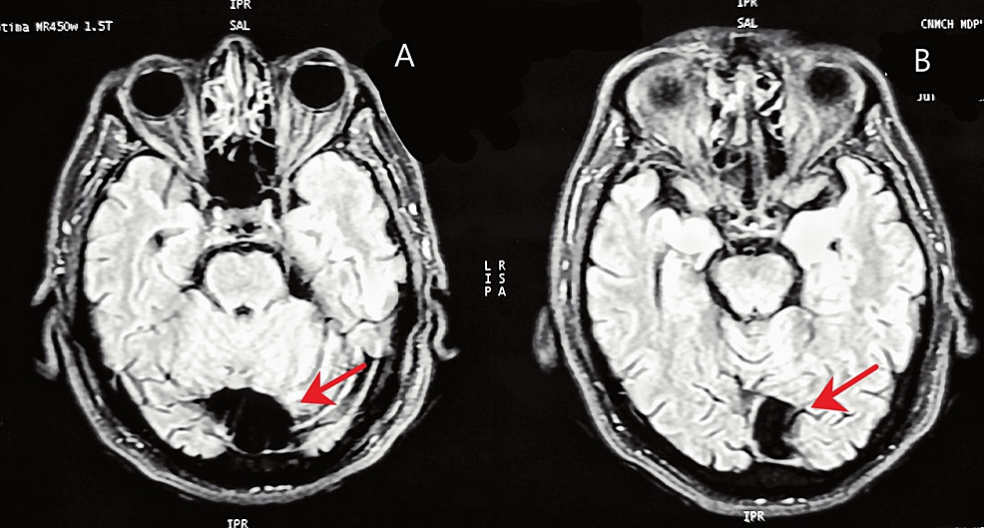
Axial sections of head MRI (T1-weighted) showing retrocerebellar arachnoid cyst
